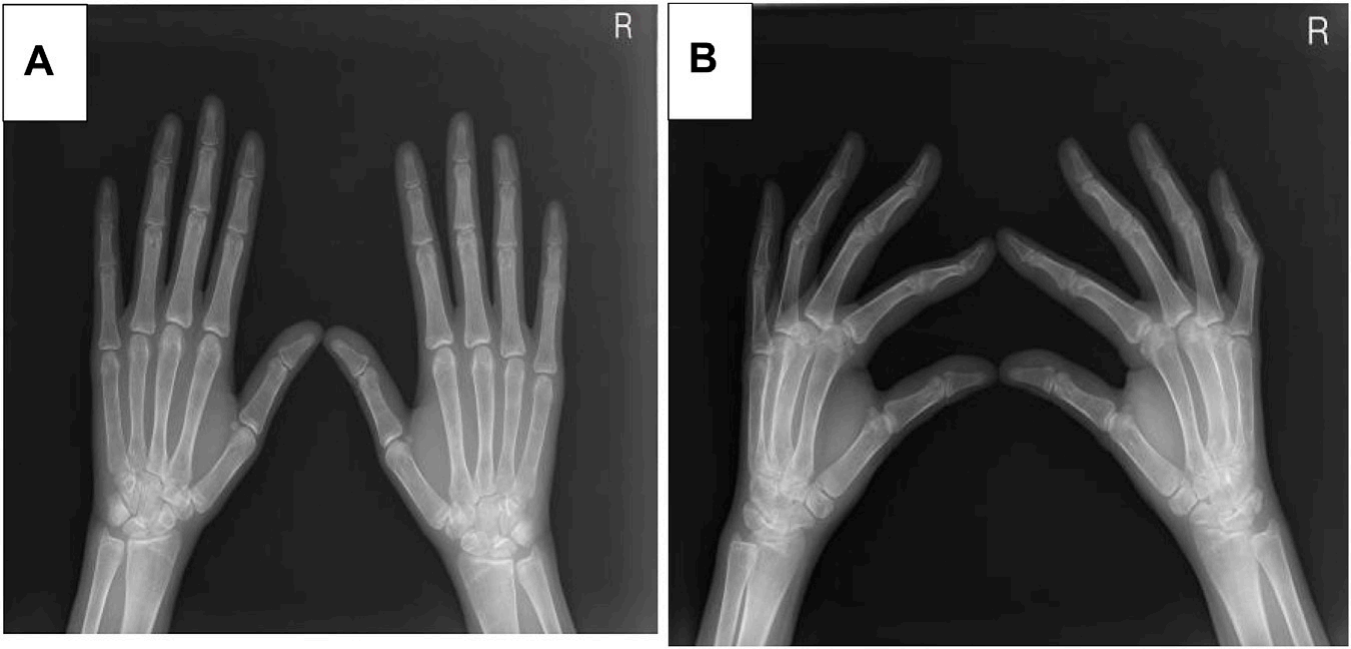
(A) The X-ray positive position of the patient. (B) The X-ray lateral position of the patient.
